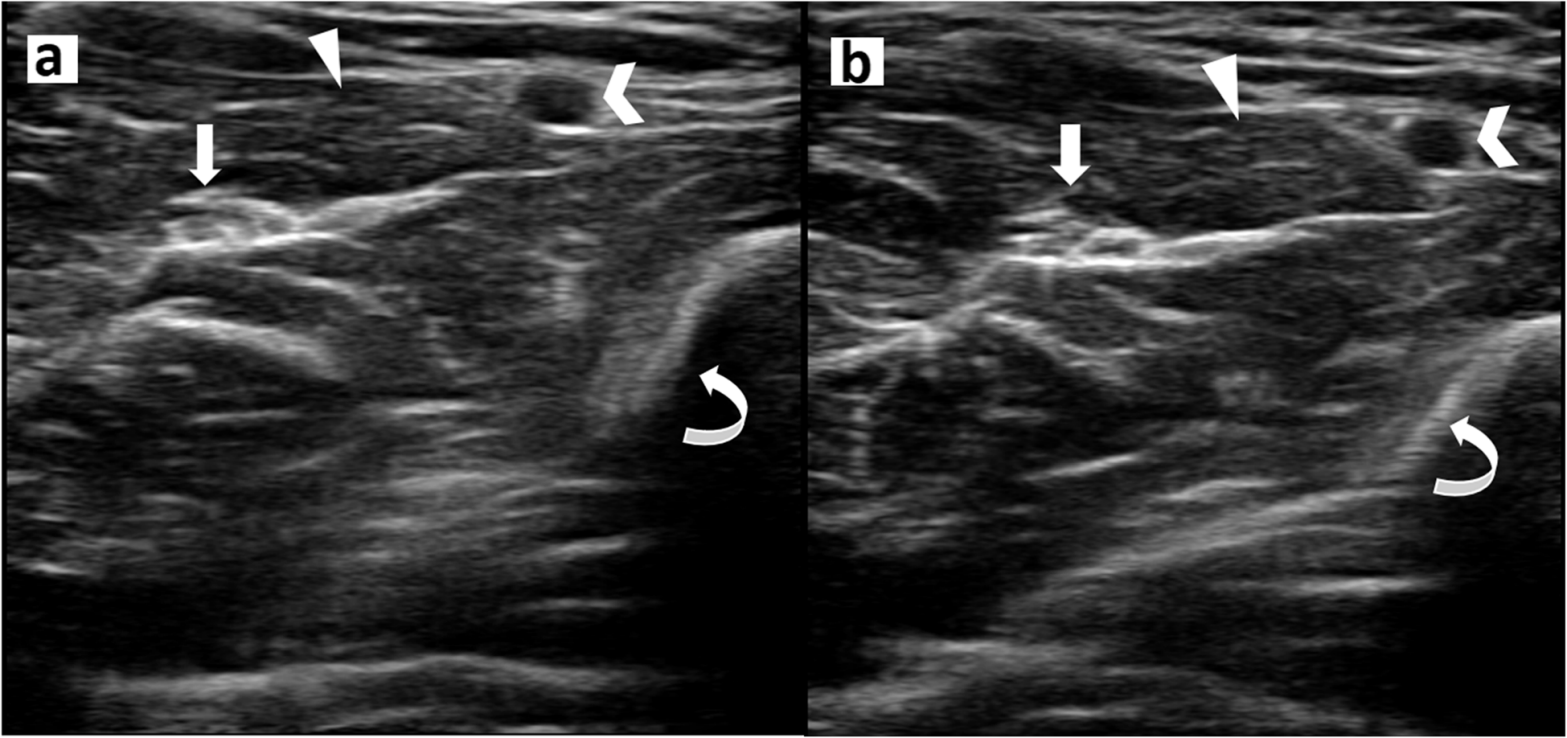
Transverse ultrasound images of the left mid forearm obtained through conventional (a) and robotic (b) techniques which demonstrate median nerve (arrow), flexor digitorum superficialis muscle (arrow head), radial artery (chevron), and radius (curved arrow)
